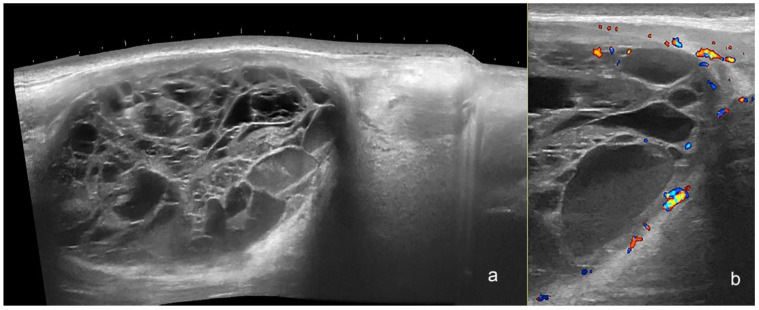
(a,b). Myxoid liposarcoma. Ultrasound images (a), panoramic greyscale longitudinal view; (b) color Doppler transverse view at the posterior aspect of the thigh presents an oval-shaped hypodermal mass with a mixed echogenicity and multiple anechoic lacunar spaces and hypoechoic areas. There is prominent hyperechogenicity compatible with edema in the adjacent hypodermis and a posterior acoustic reinforcement below the mass. On color Doppler, there is increased vascularity in the periphery of the structure.
